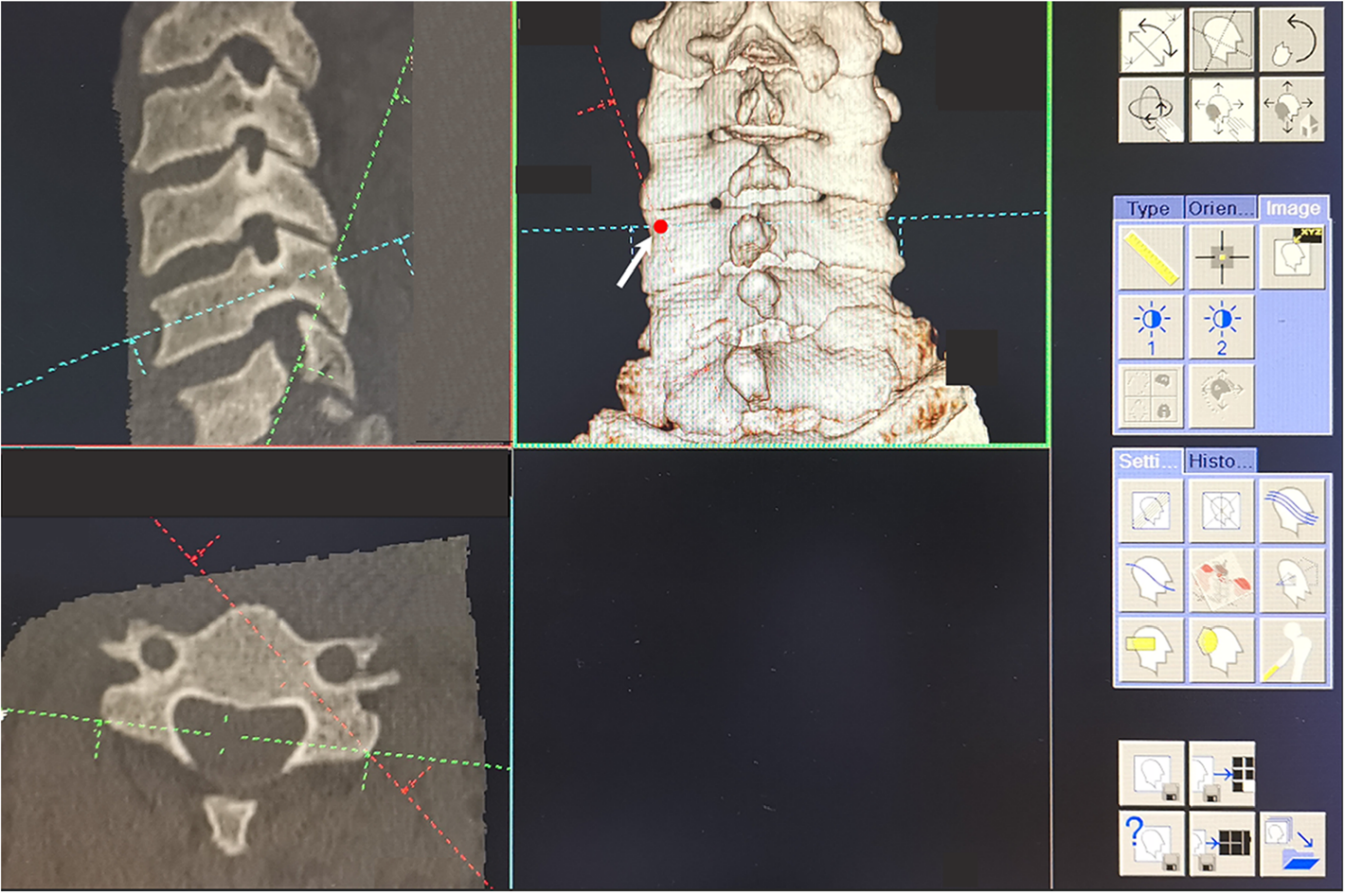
The ideal pedicle trajectory was defined as a line passing through the centre of the pedicle on the transverse and sagittal CT images (left column). The three-dimensional cervical model was reconstructed using cervical spine transverse CT images. On the three-dimensional reconstructed cervical model, the ideal pedicle trajectory would penetrate the posterior surface of the lateral mass, and this point was then identified as the optimal entry point passing through the centre of the pedicle (right column)
